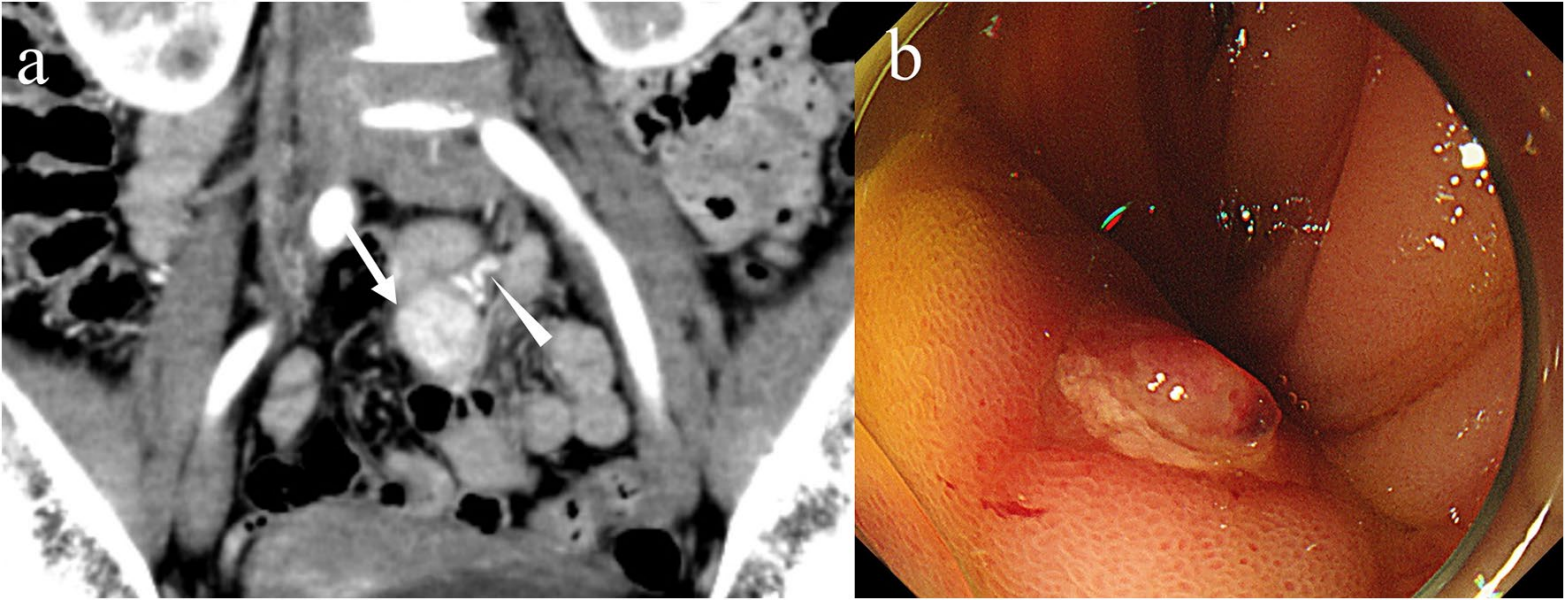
Gastrointestinal bleeding caused by a gastrointestinal stromal tumor in the small bowel. A 59-year-old female visited an emergency department with a continuous tarry stool after hematochezia. Her hemoglobin level decreased from 12.0 g/dL to 8.3 g/dl for a day. Contrast-enhanced CT showing a hypervascular tumor in the small intestine (a: arrow). The enlarged vein continuing to the tumor is markedly enhanced in the arterial phase (a: arrowhead). Double balloon endoscopy showing a submucosal tumor with ulcer (b). Inoue A. Gastrointestinal stromal tumor (GIST). (In Mizunuma K, et al., eds. *Diagnosis Imaging and Intervention Therapy of Abdominal Emergencies*. Tokyo: MedicalView; 2018. P200, Fig. 1
